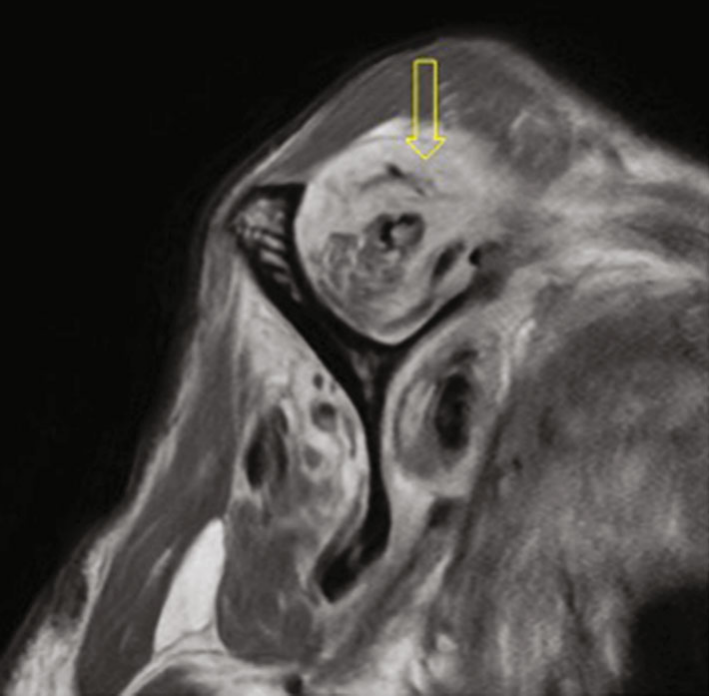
Sagittal T2-weighted MRI; the yellow arrow demonstrates atrophy and fatty infiltration of the right supraspinatus muscle, consistent with a chronic rotator cuff tear.
